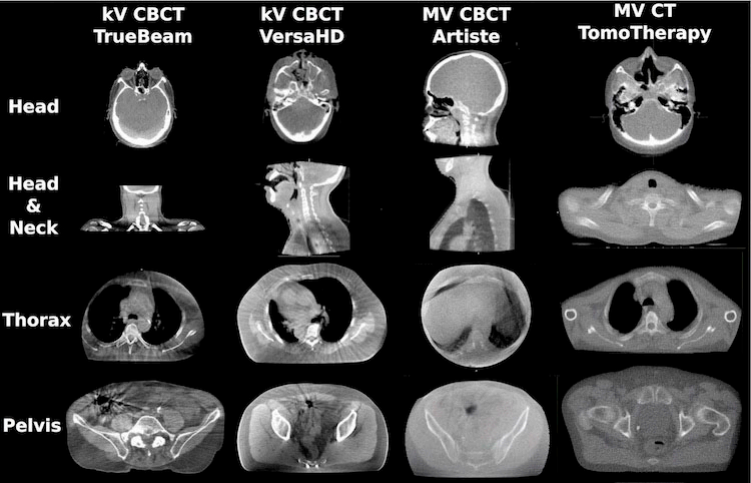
Patient CT images of different treatment sites. The images were acquired using the indicated machines’ on‐board imaging system.
